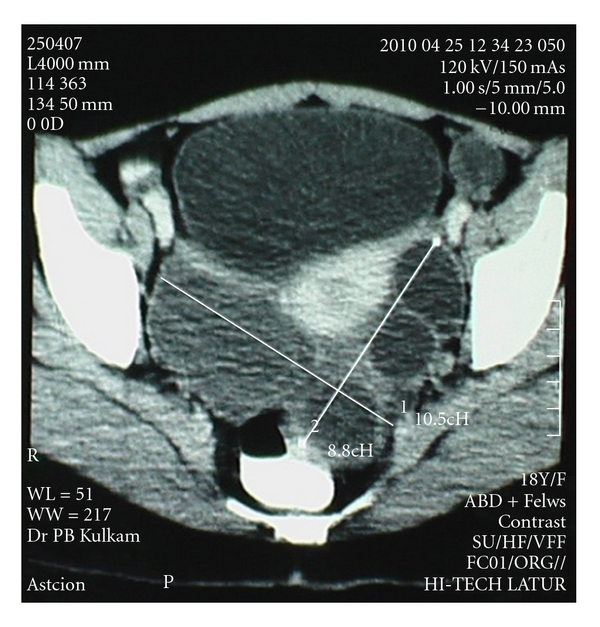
CT Scan showing hydatid cysts in the pelvis.
